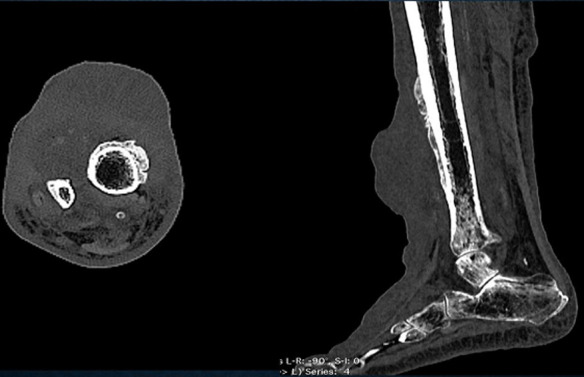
computerised tomography (CT) axial and sagittal reconstruction images of the same patient shows areas of soft tissue edema, heterogeneous linear densities and few areas of faint calcification in the region of the lobulated skin lesion; thick unicortical periosteal reaction of the tibia for a, short segment; adjacent to the lesion is noted
